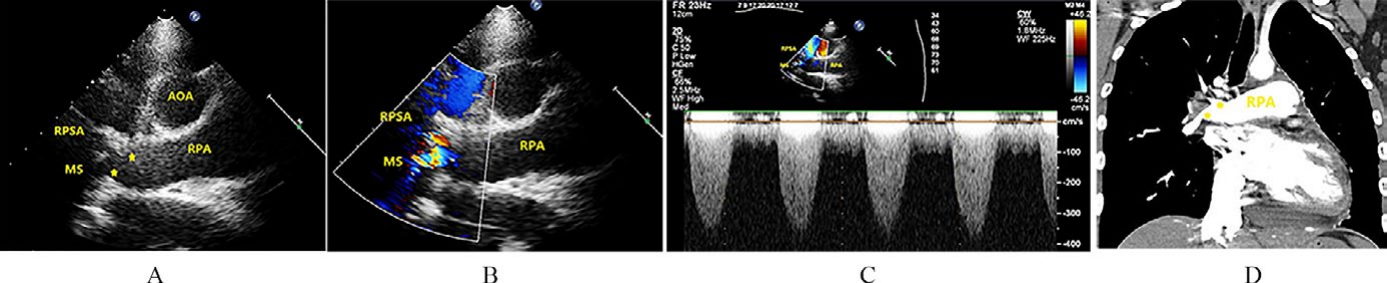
A. Suprasternal right pulmonary artery long‐axis view showed right pulmonary artery intimal thickening in RPSA and middle segment of right pulmonary artery (movie clip S3). B. CDFI showed multicolored mosaic flow signals in RPSA and middle segment of right pulmonary artery. C. CW showed high velocity of blood flow in the middle segment of right pulmonary artery. D. Coronal view by CTPA showed RPSA and RPIA narrowing (asterisk *). AOA = aortic arch; RPA = right pulmonary artery; RPSA = right pulmonary superior artery; MS of RPA = medial segment of the right pulmonary artery; RPIA = right pulmonary inferior artery; CDFI = color Doppler flow imaging; CW = continuous wave; CTPA = computed tomographic pulmonary angiography
